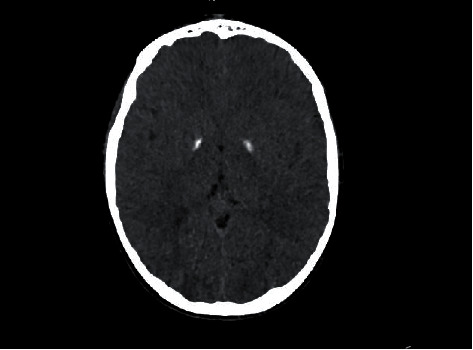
Axial CT scan showing bilateral calcifications of the globus pallidus.
